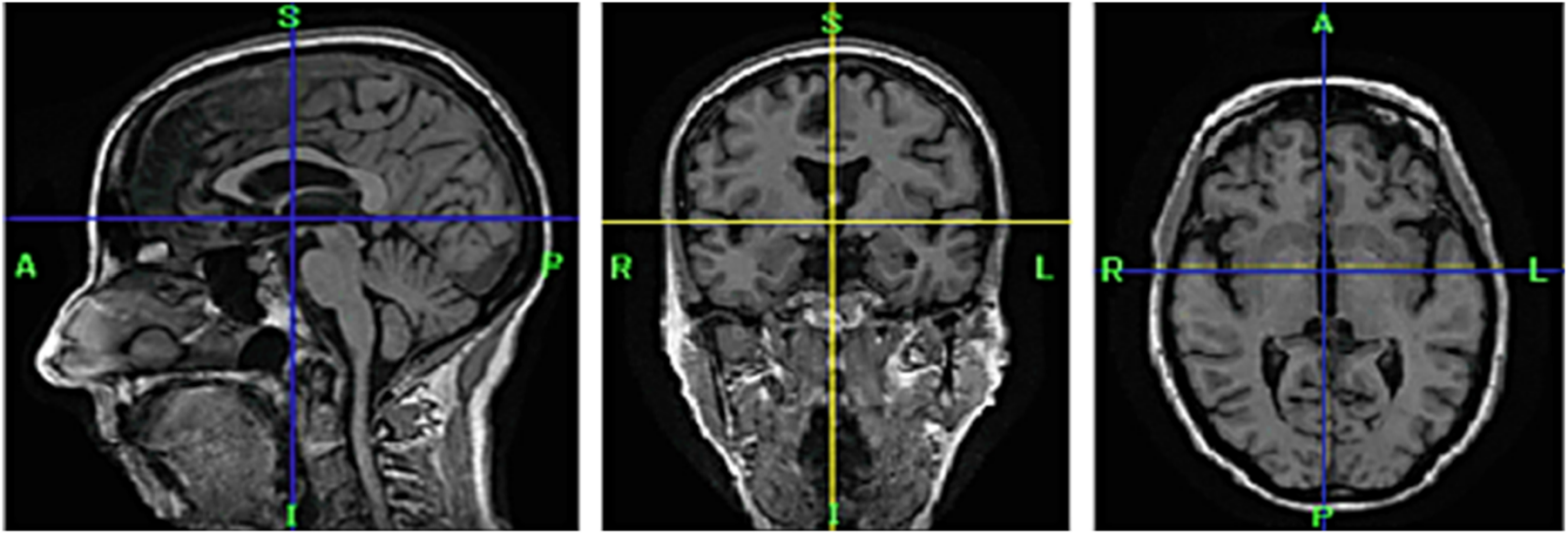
A three‐dimensional brain magnetic resonance imaging shows three planes (sagittal, coronal, and axial) from left to right.^13^
